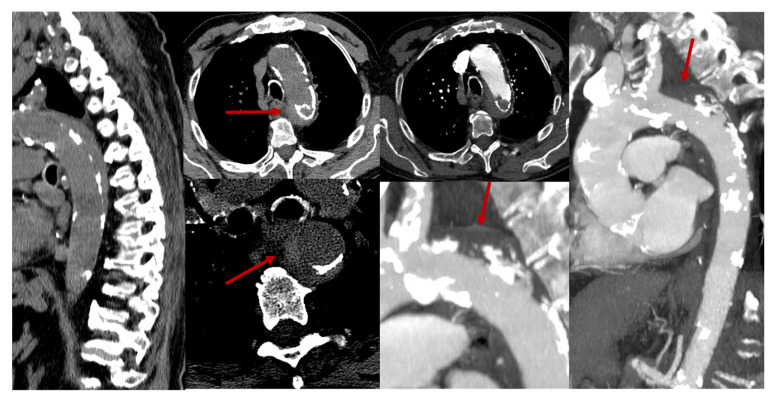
An 80-year-old patient reported severe acute back pain, followed by immediate onset of paraplegia with sensory level at D3-D4. An emergent CT angiography of the thoracic and abdominal aorta diagnosed an aortic dissection. Even in the pre-contrast sagittal reconstruction scan, an eccentric, crescent-shaped, isodense thickening was clearly visible in the wall of the thoracic aorta, just after the origin of the left subclavian artery, delineated by coarse calcifications on the intimal side of the aortic wall, highly suggestive of a mural hematoma. In the axial scans, a slightly hyperdense component was also outlined, possibly indicating ongoing bleeding. The post-contrast study confirmed the finding, with better delineation of the endoluminal dissection of severe and complicated aortic atheromatosis, where the fracture of the fibrous cap (in the segment without calcifications) could have created the entry point for the formation of the mural hematoma (red arrows).
